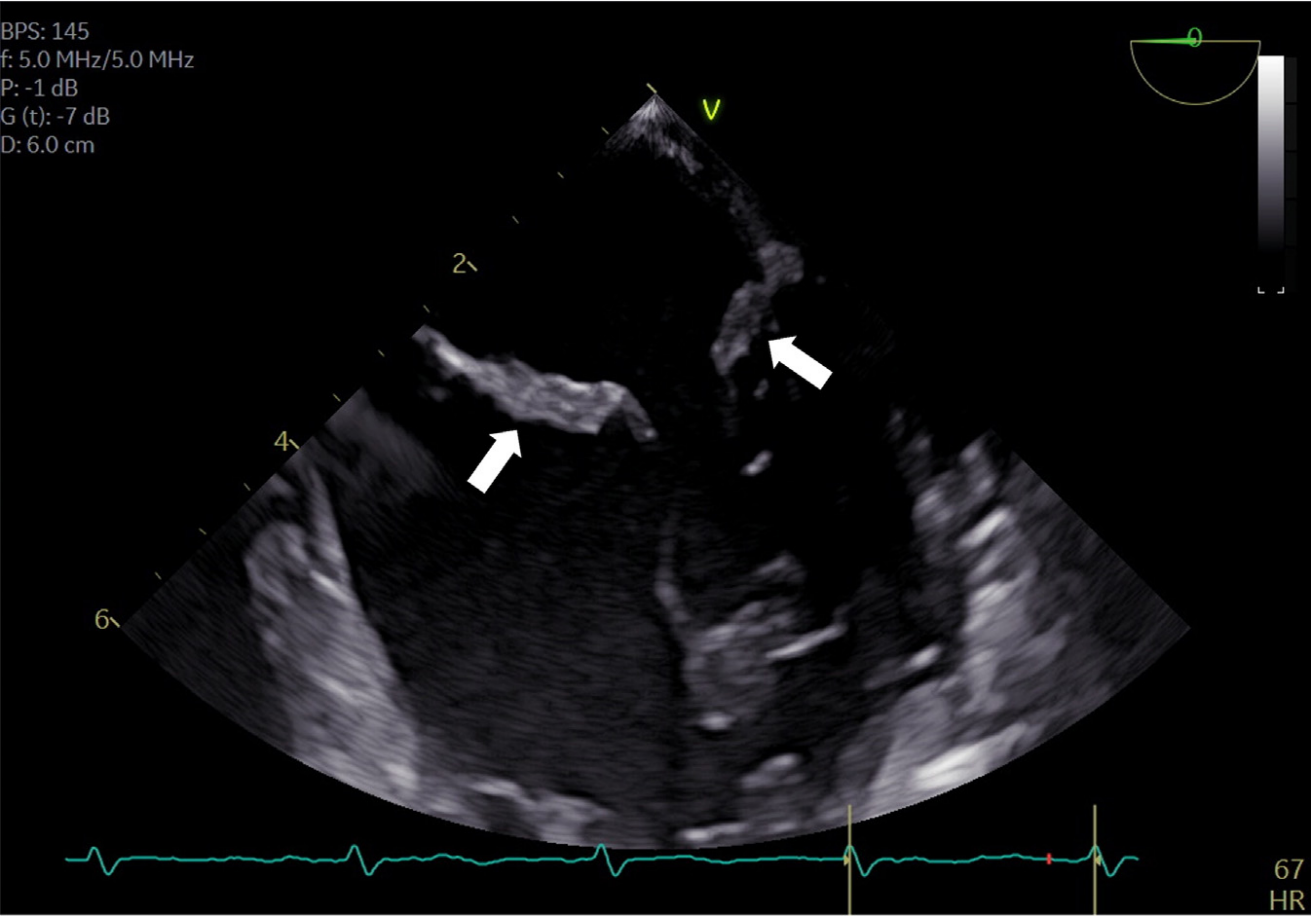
Transesophageal Echocardiogram Transesophageal echocardiography showing vegetations and destruction of the anterior and posterior MV leaflet (20 mm x 13 mm).
